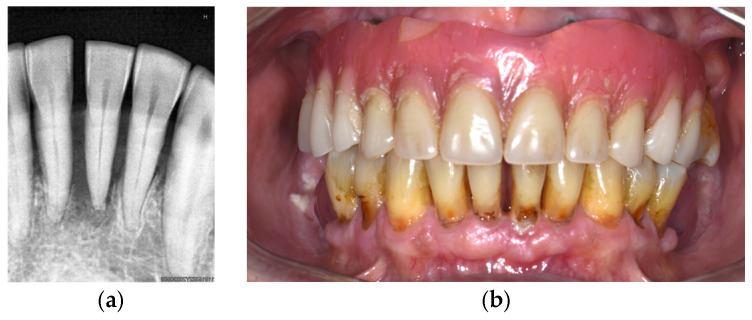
(a) Periapical radiograph of a lower incisor (3.1) with periapical process and one-wall intrabody defect. (b) Generalized periodontitis stage IV. Localized suppuration at tooth 3.1.
